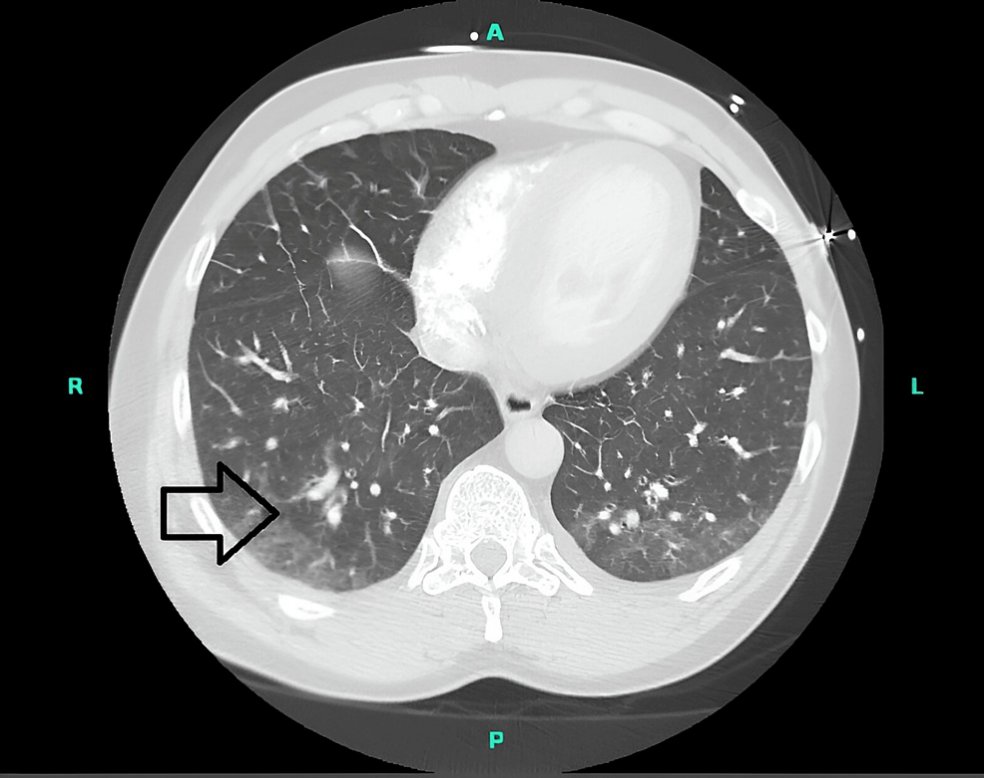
CT chest on admission CT showed mild alveolar opacities concerning pulmonary edema or pneumonia and emphysema (black arrow)
